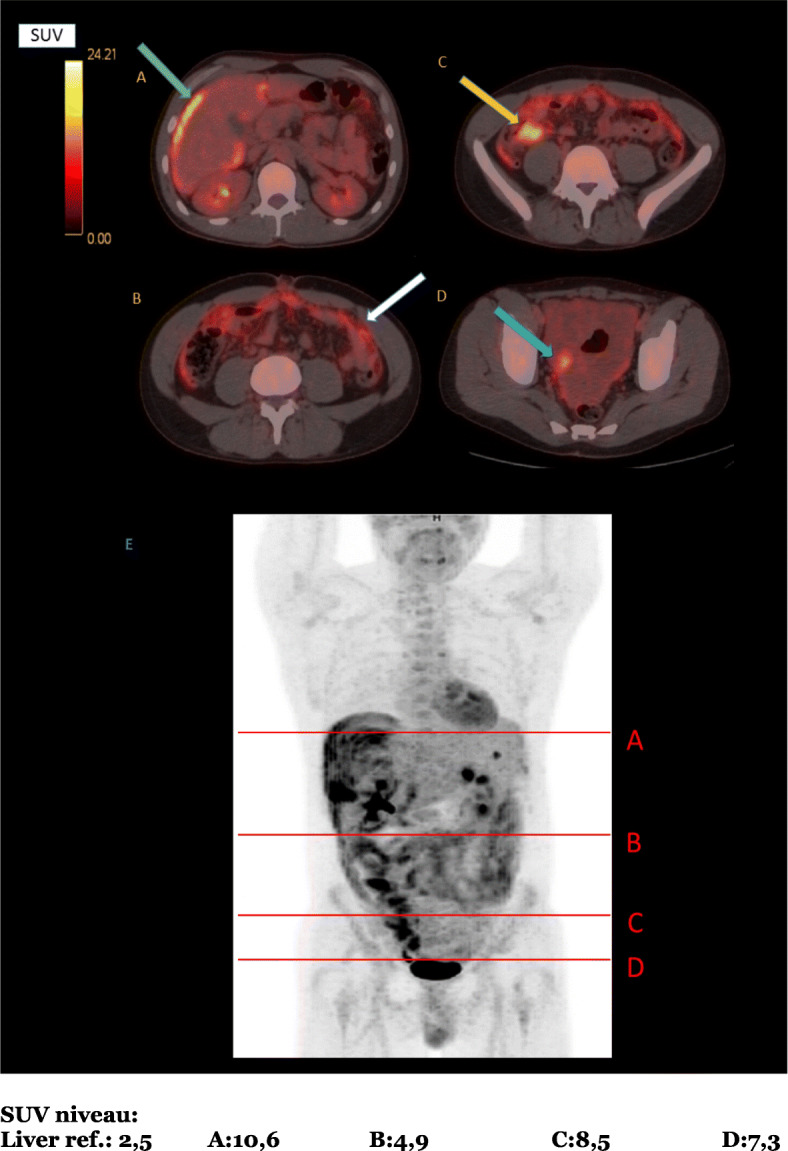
FDG-PET/CT scans showing foci with pathologic FDG-uptake. (a) Surface of the liver (green arrow). (b) Omental uptake (white arrow). (c) Colonic uptake (yellow arrow). (d) Pelvic ascites (blue arrow). (e) Levels of the images shown in (a)–(d) (FDG standardized uptake value scale shown)
